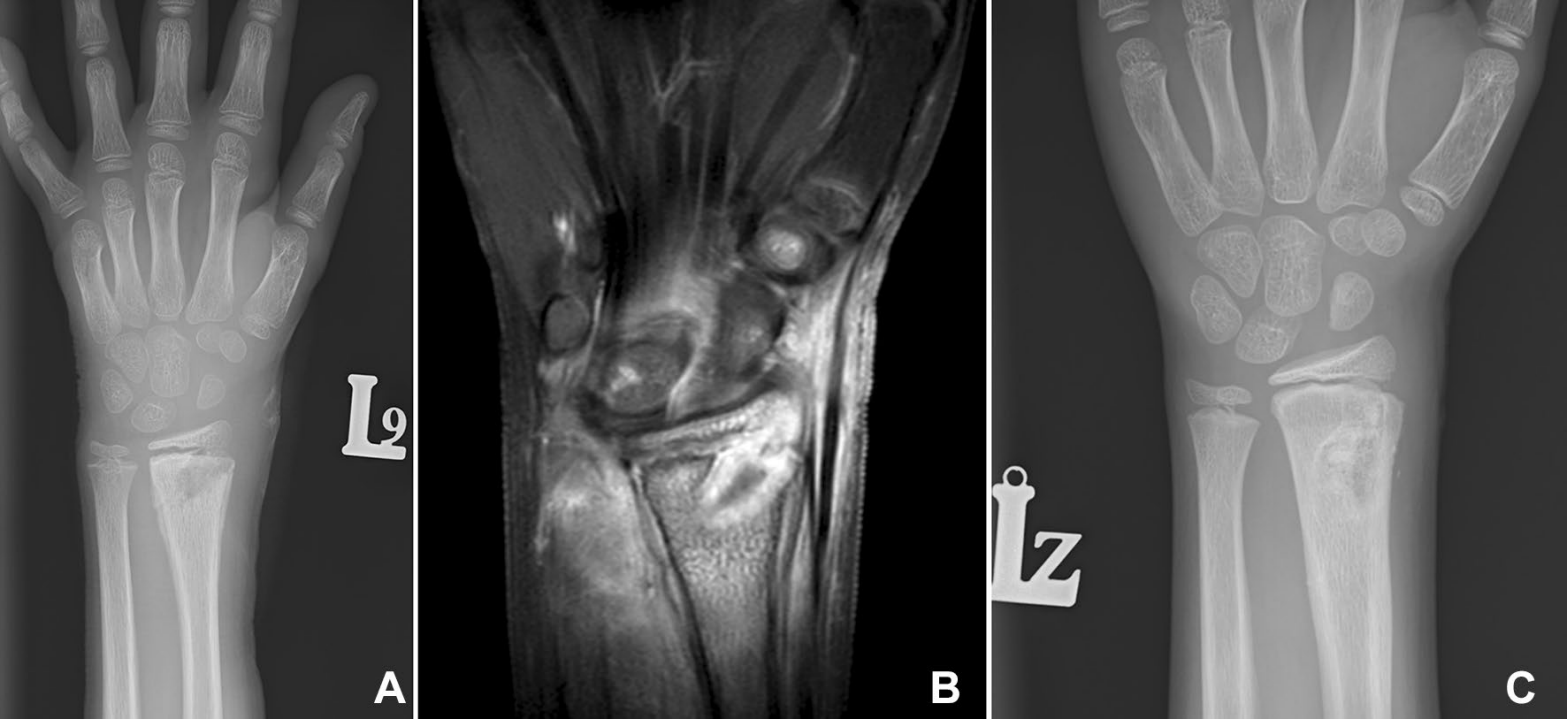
Bone osteolysis along the pin track (Dahl grade 4) X-ray (A), magnetic resonance (B) and X-ray after successful Masquelet procedure (C)
